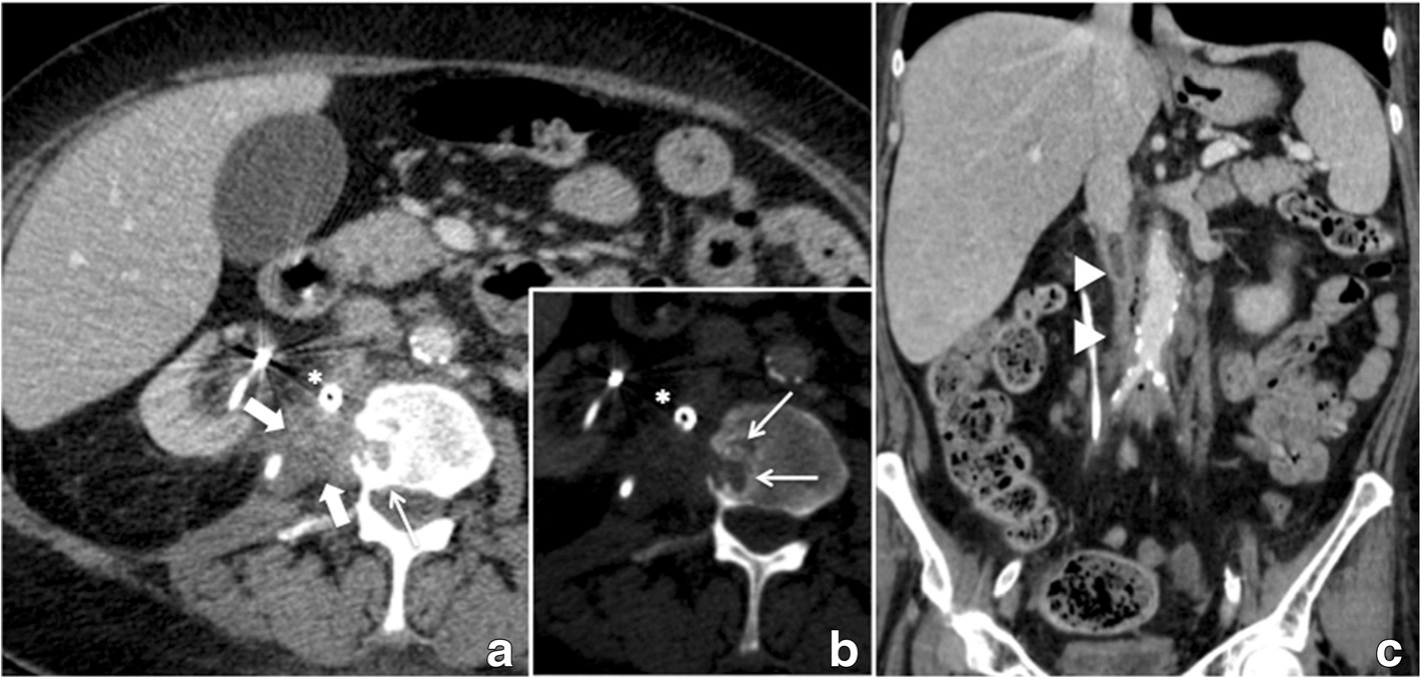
Initial follow-up CT scan 2 months after treatment with percutaneous drainage showing a marked decrease in size of the abscess (thick white arrow; a), however, Osteolytic bone lesion in adjacent second lumbar vertebra (small white arrows a and b), a streaky densification of the pericaval connective tissue and a nearly complete thrombotic occlusion of the infrarenal inferior vena cava (white arrowhead c)), which was probably infected (small gas bubbles). Infection related air bubbles in the retroperitoneal space can also be seen now along the aorta to below the aortic bifurcation
